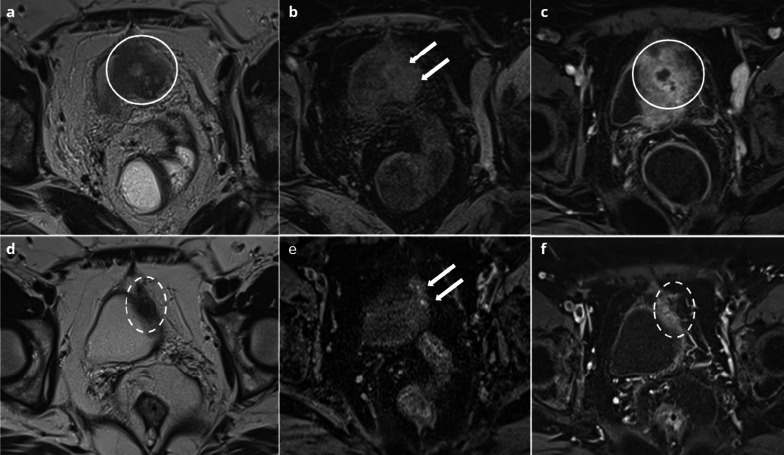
48-year-old female, with a history of severe endometriosis treated by surgery, presenting with hypogastric pain, elevated C-reactive protein (CRP) and white blood cell count. Axial T2W sequence (a) shows a complex cystic mass of the bladder wall (circle). b No hemorrhagic signal is observed on axial T1FSW sequence (arrows). After injection, axial T1FSW (c) depicts perivesical fat stranding and enhancement consistent with an inflammatory process (circle). Infected bladder endometriosis was suspected given the patient’s history, and a follow-up MRI was performed after antibiotherapy. Axial T2W sequence after treatment (d) shows a decrease in size of the mass. e Hyperintense spots appear on T1W FS sequences (arrows), and (f) inflammatory signs decrease after injection (dotted circle), consistent with the resolution of an infectious process involving the endometriotic mass
